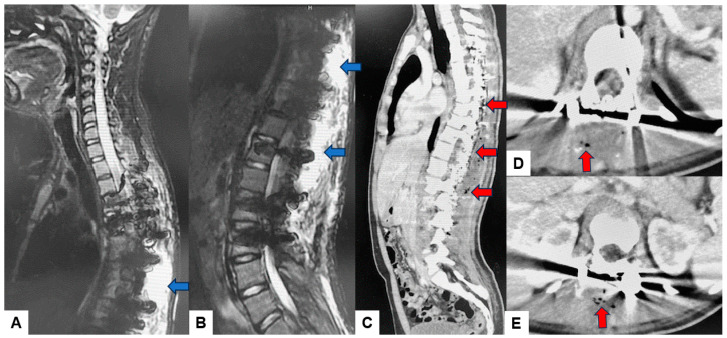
13 M, Adolescent idiopathic scoliosis, MRI and enhanced CT at postoperative Day 12. (A) Midsagittal cervicothoracic T2 weighted MR imaging, (B) Midsagittal thoracolumbar T2 weighted MR imaging, (C) Enhanced midsagittal reconstruction CT, (D) Enhanced T8 axial CT, (E) Enhanced L1 axial CT. Blue arrows indicate postoperative abscess/effusion. Red arrows revealed gas inside the abscess/effusion.
